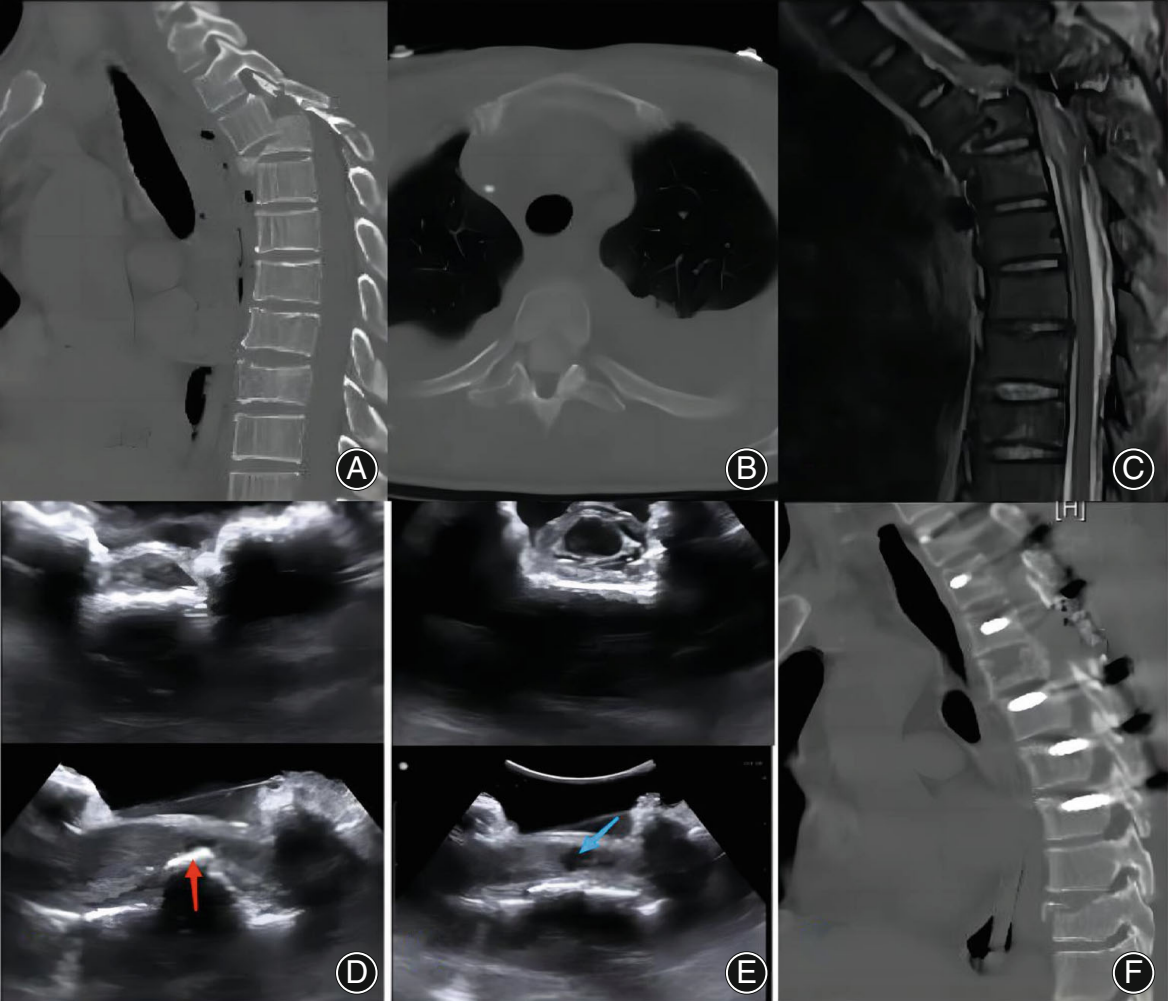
Case 3: Computed tomography (CT) and magnetic resonance imaging showed T3/4 fracture and dislocation, T9 compression fracture, and obvious spinal canal encroachment (A–C). The surgeon used intraoperative ultrasound in lateral decubitus position and observed swelling and thickening of the spinal cord at the fracture site, and found displaced bone segments compressing the spinal cord (D red arrow). After further decompression, it was observed that the spinal cord decompression was completed (E), and a large hypoechoic area (E, blue arrow) in the spinal cord was found. Postoperative CT showed satisfactory canal decompression and fracture reduction and good internal fixation position (F).
